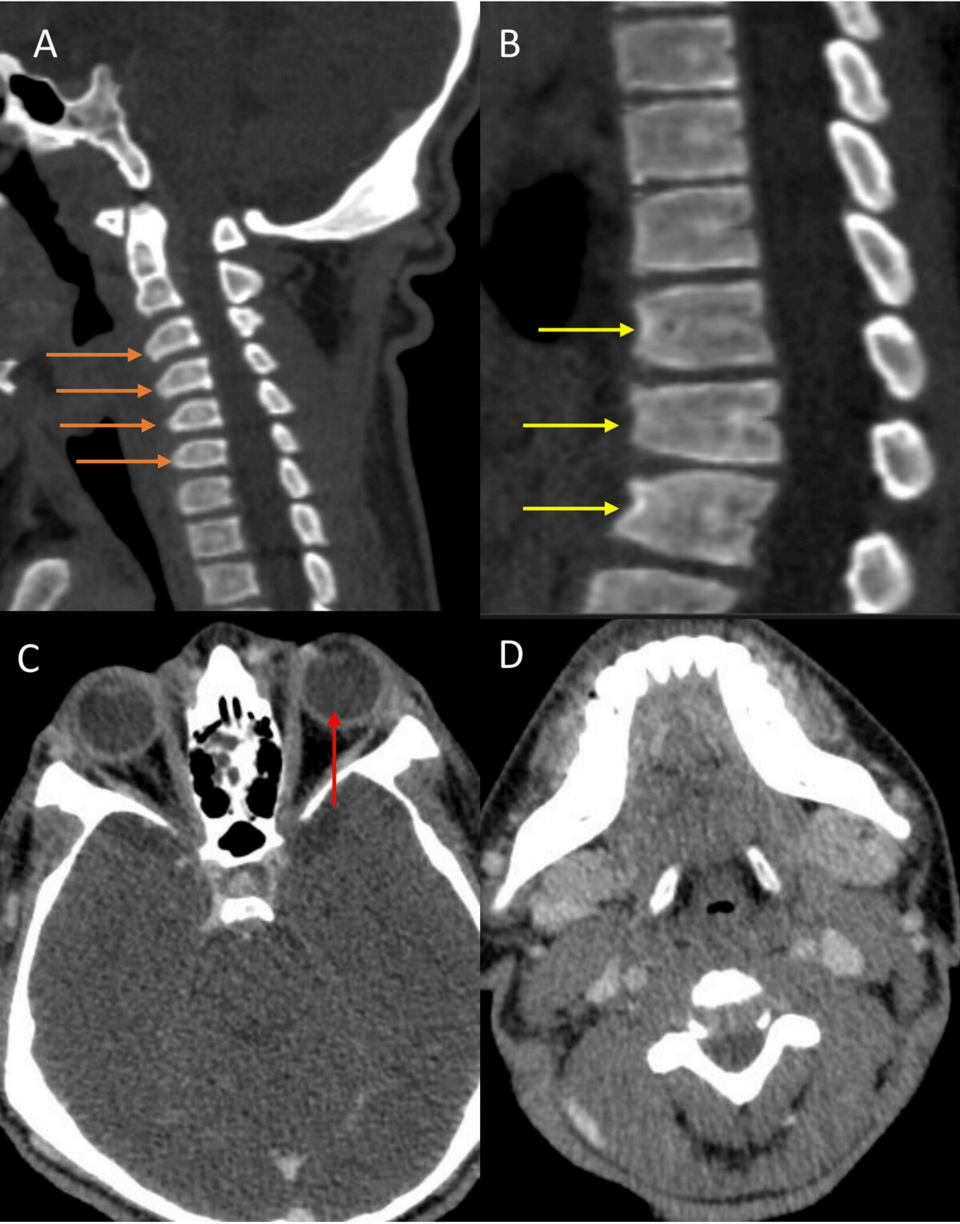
The CT sagittal view shows (A) the abnormal bullet shape of cervical vertebrae (orange arrows) causing spinal compromise and (B) the abnormal shape of thoracic vertebrae (yellow arrows). The axial CT section of the brain reveals (C) hypertelorism (red arrow) and (D) abnormal craniofacial bones with a short body of the mandible.
